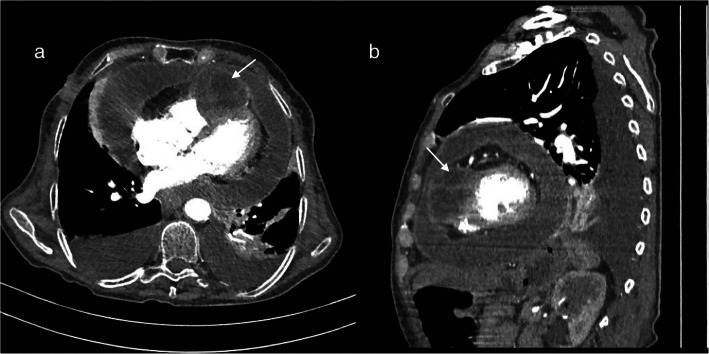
Appearance of massive pericardial effusion with hydatid cyst compressing the right ventricle (arrows) at CT scan. a Transversal view. b Sagittal view
